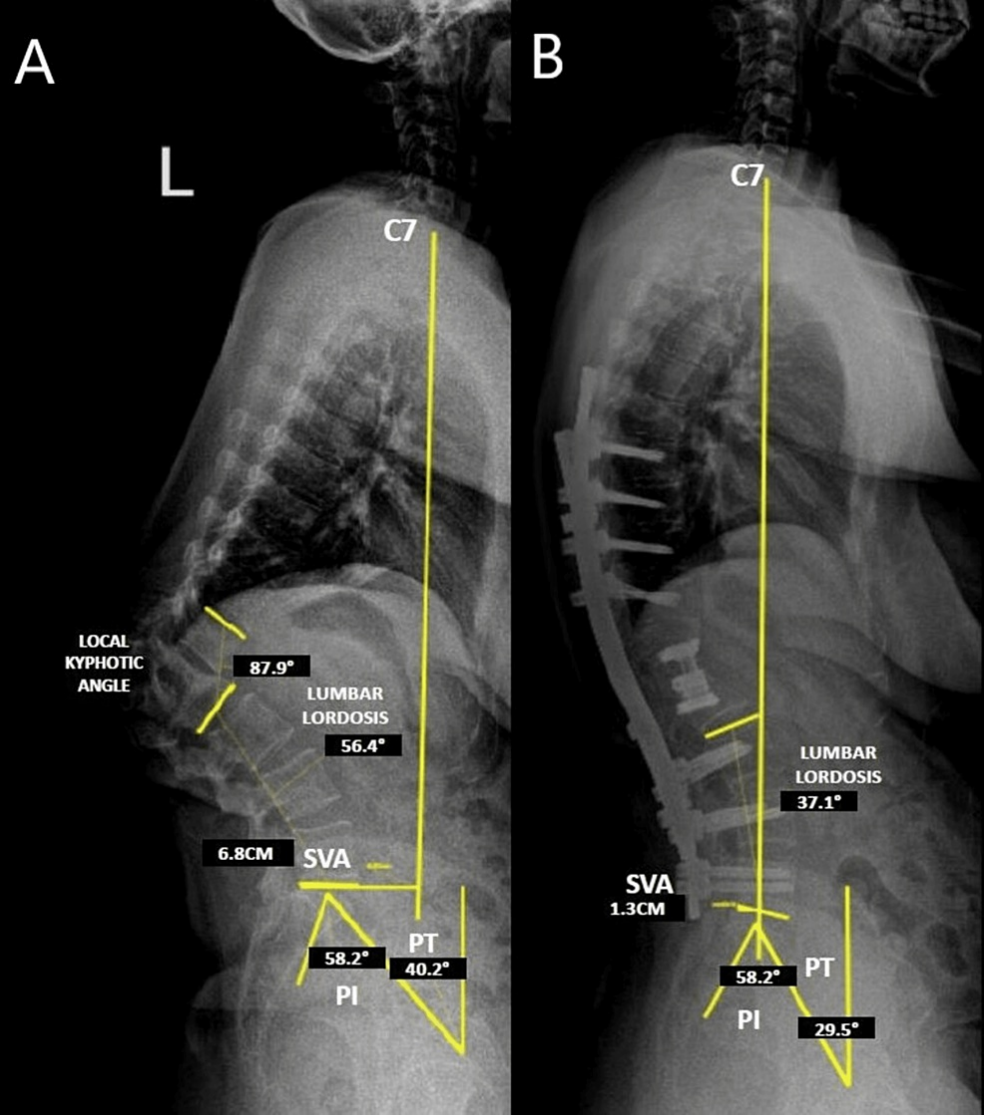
Preoperative (A) and postoperative (B) lateral whole-spine radiography for sagittal balance parameters.
